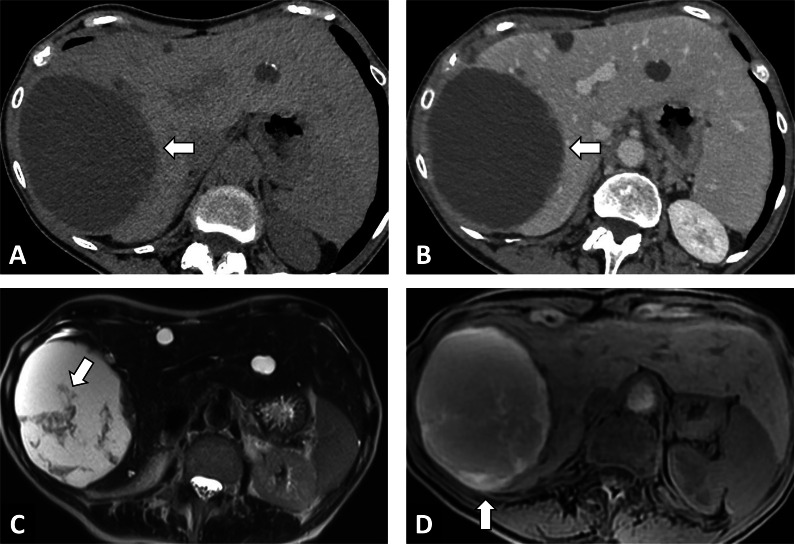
Differential diagnosis of a cystic echinococcosis lesion in a 78-year-old woman. A Unenhanced CT image in the axial plane showing a right liver cyst. B CT image in the axial plane at the portal phase showing a cystic lesion of the right liver with thickening wall. C T2-weighted MR image in the axial plane showing heterogeneous content without daughter cysts. D T1-weighted MR image in the axial plane showing hyperintense signal of the liver cyst confirming the diagnosis of hemorrhagic liver cyst
